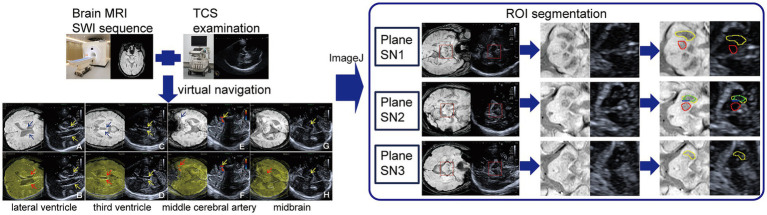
The workflow of TCS-MR fusion imaging, including representative planes of superimposition, and ROI segmentation. Left panel: the blue and yellow arrows indicated the lateral ventricle body (A,B), the third ventricle (C,D), and the left middle cerebral artery (E,F) on MRI and TCS, respectively, which were perfectly overlaid in fusion mode (red arrows). The yellow and red arrows in G,H indicated right substantia nigra hyperechogenicity (SNH) on TCS and fusion mode, respectively. Right panel: the yellow, red, green, and blue ROIs indicated boundaries of substantia nigra (SN), red nucleus, as well as SNH in the SN and dorsal bound of the SN, respectively. MRI, magnetic resonance imaging; SWI, susceptibility-weighted imaging; TCS, transcranial sonography; ROI, region of interest; plane SN1, the plane with the largest area of red nucleus on MRI; plane SN2, the plane with the largest area of substantia nigra hyperechogenicity; plane SN3, the plane where the red nucleus is barely identified or no longer visible.
